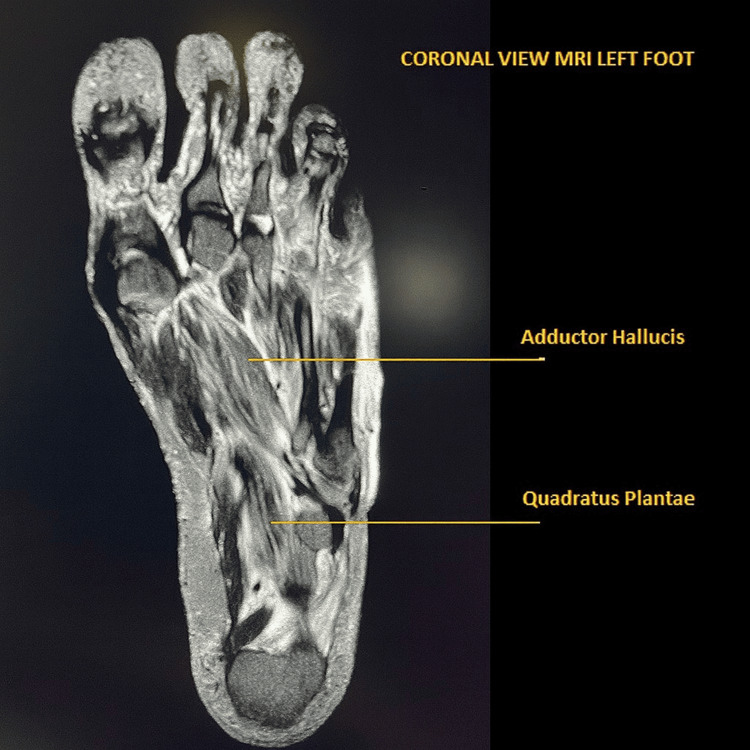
Coronal view MRI of left foot showing hyperintensity in the adductor hallucis and quadratus plantae muscles
